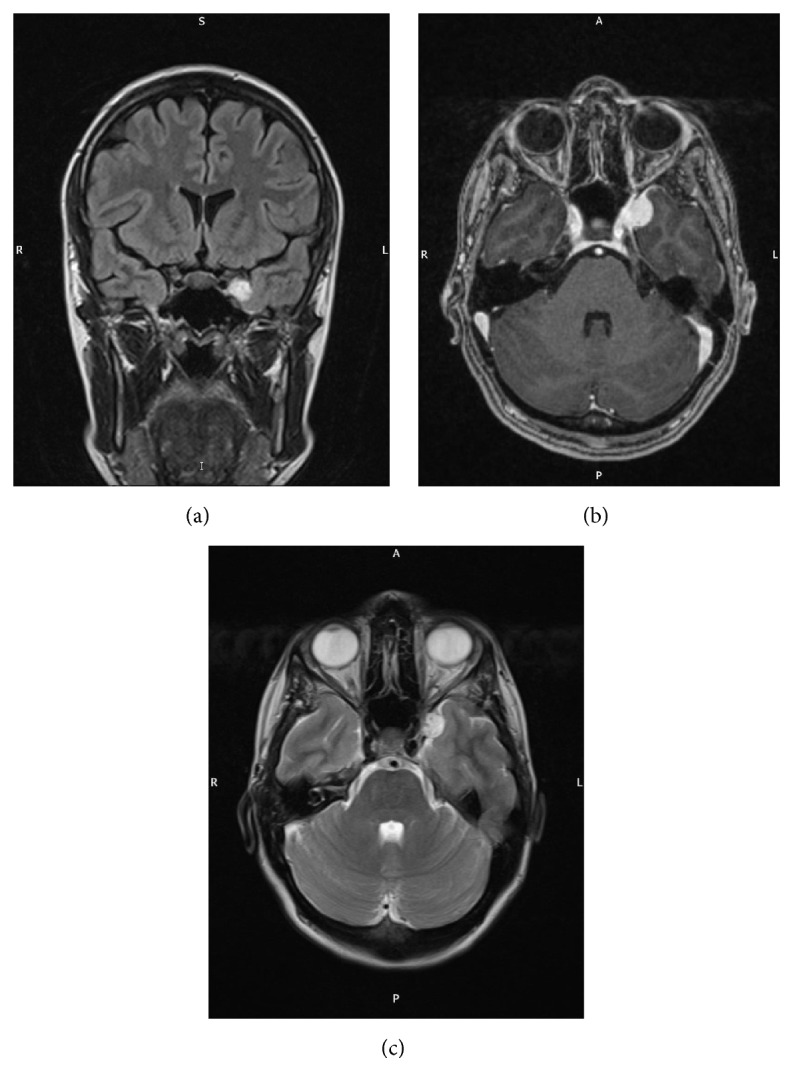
MRI images of a left sided lateral wall cavernous sinus meningioma coronal flair (a), T1 with contrast (b), and T2 axial (c).
